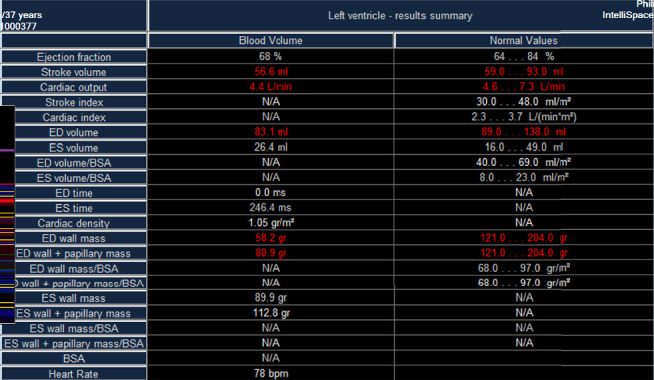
No obvious abnormal changes such as myocardial edema as indicated by the cardiac magnetic resonance imaging for Case 1.
